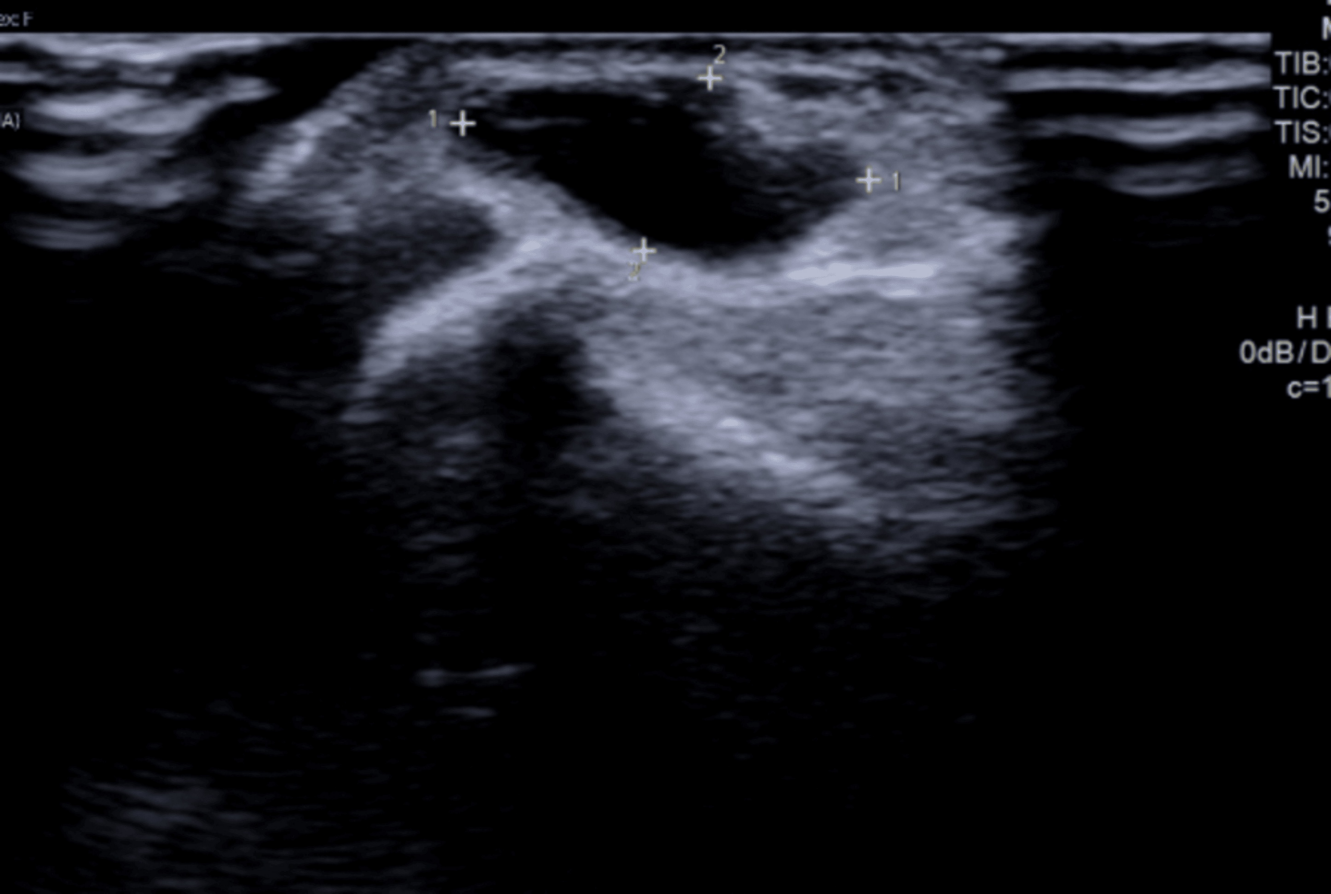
Sonographic findings of the volar ganglion of the wrist.
